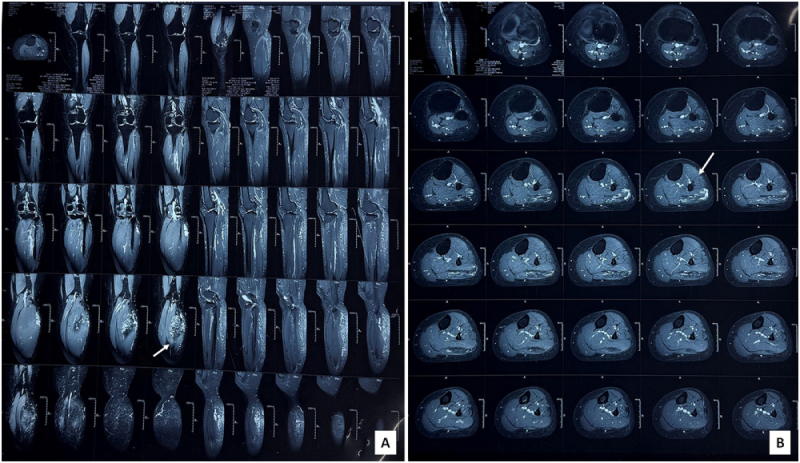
(A) Coronal and sagittal MRI images showing a serpiginous cluster of vessels with fatty tissue in the superficial aspect of the proximal gastrocnemius lateralis muscle (arrow), with mild extension into the adjacent soleus muscle. (B) Axial T2-weighted MRI image demonstrating the vascular lesion with heterogeneous signal intensity and draining branches into the short saphenous vein (arrow).
